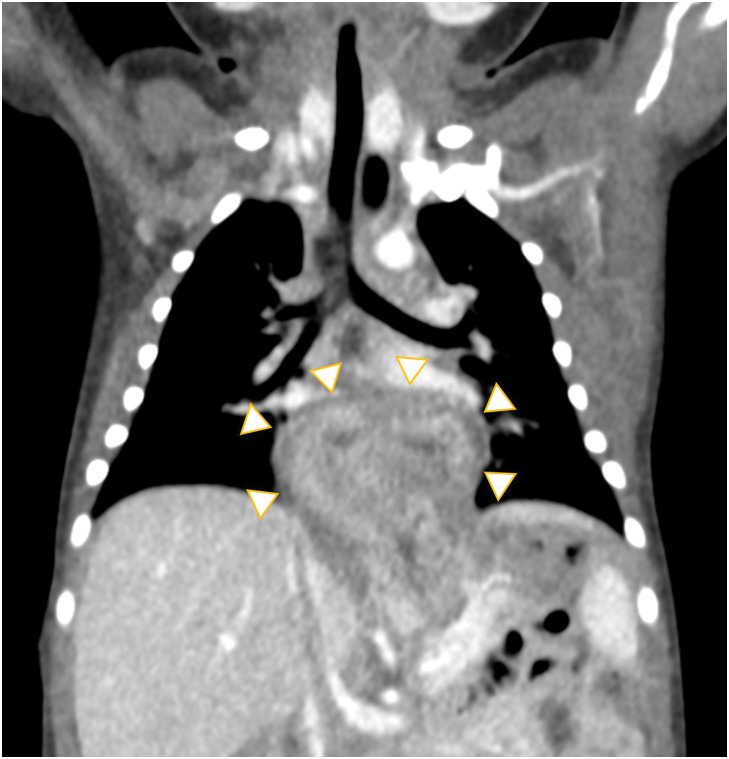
CT. The upper half of the stomach protruded into the inferior mediastinum, appearing as a dome-shaped mass (arrowheads).
